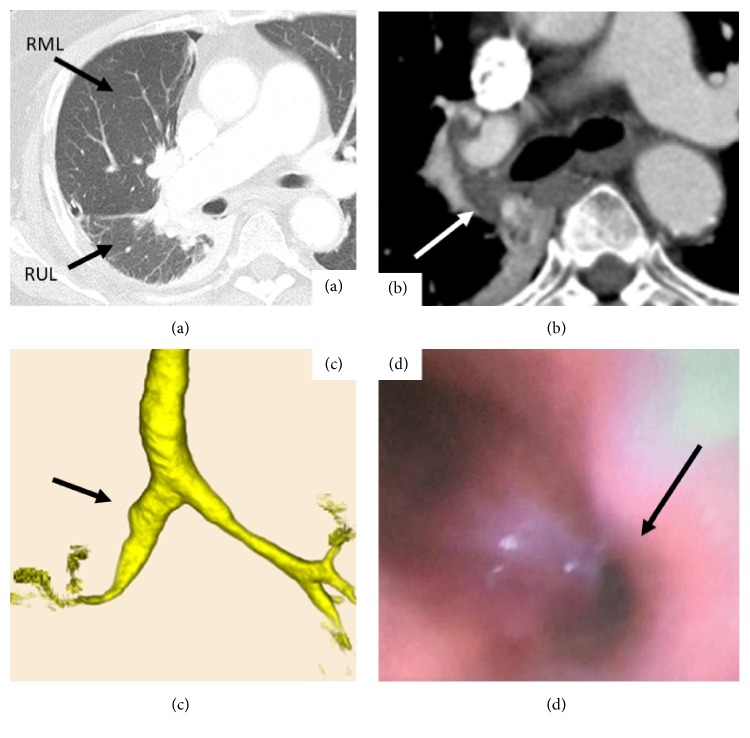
(a) An emergency chest computed tomography (CT) showed the middle lung lobe had been pushed upward and forward, and the right upper lung lobe had twisted dorsally. (b) The right upper bronchus with hilar peribronchovascular soft tissue edema (arrow). (c) Three-dimensional CT scan of bronchial stenosis (arrow). (d) Emergency bronchoscopy showing severe right upper bronchial stenosis (arrow). RUL: right lower lobe; RML: right middle lobe.
